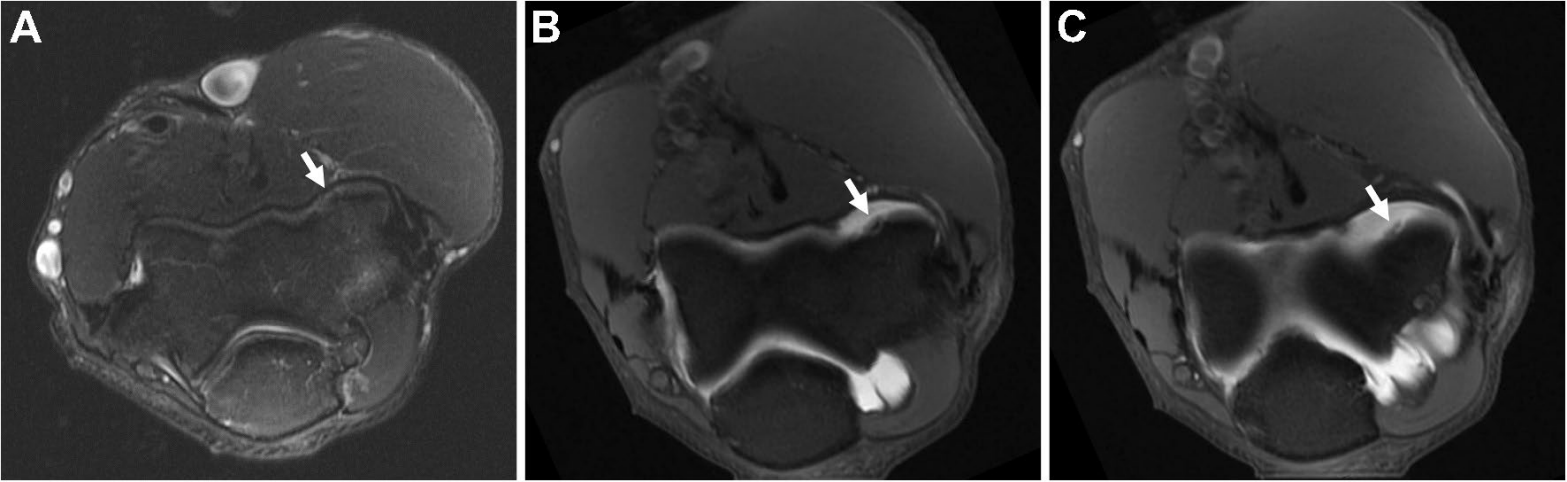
Osteochondral lesion. Thirty-eight-year-old man with elbow pain. A Axial T2-weighted fat-suppressed conventional 1.5 T MR image shows a small osteochondral lesion at the anterior capitellum, without signs of instability (arrow). B, C Axial T1-weighted fat-suppressed 1.5 T MR arthrogram images show contrast undermining the lesion, consistent with an unstable in situ osteochondral fragment
